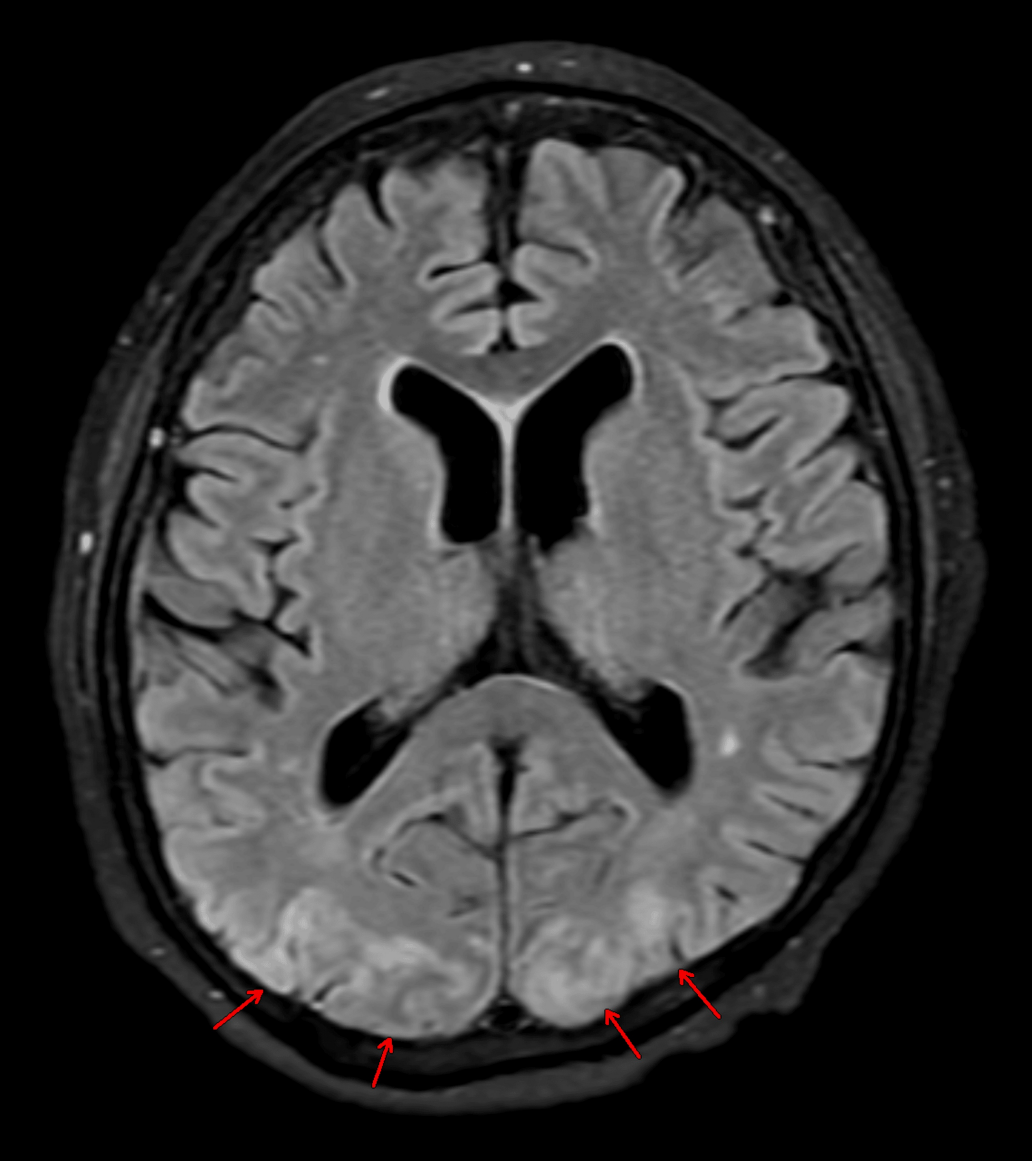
Hyperintensities on FLAIR Finding: Axial non-contrast FLAIR MRI brain showing symmetric hyperintensity (red arrow) in bilateral occipital lobes with effacement of adjacent cortical sulci. Technique: 1.5 T MRI, FLAIR sequence. FLAIR: fluid-attenuated inversion recovery; MRI: magnetic resonance imaging
